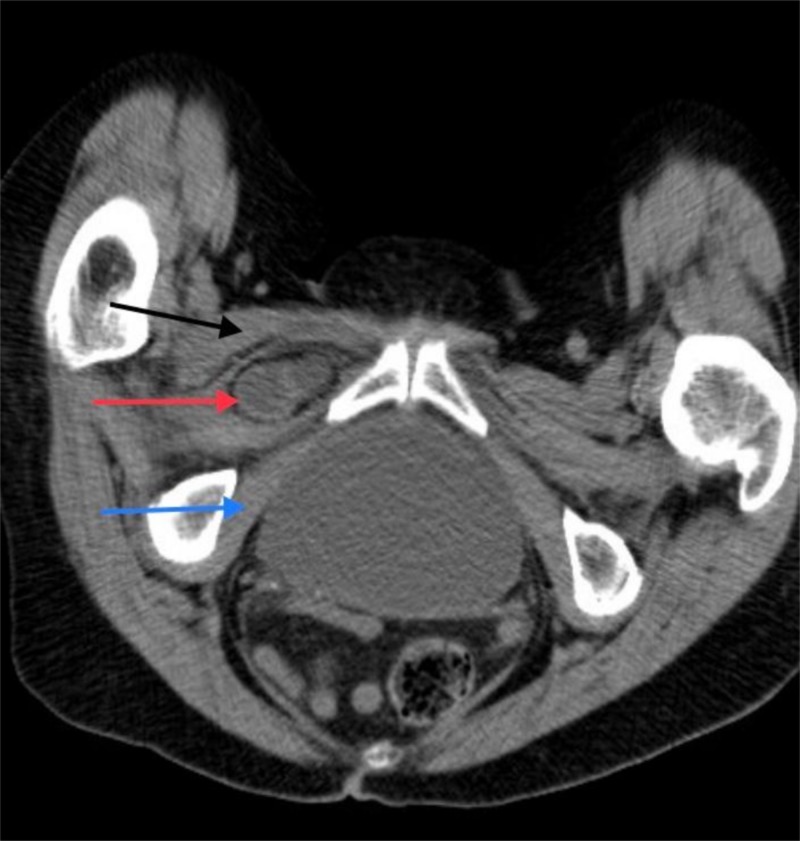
CT scan pelvis Pectineus muscle (black arrow); small bowel (red arrow); obturator muscle (blue arrow)
